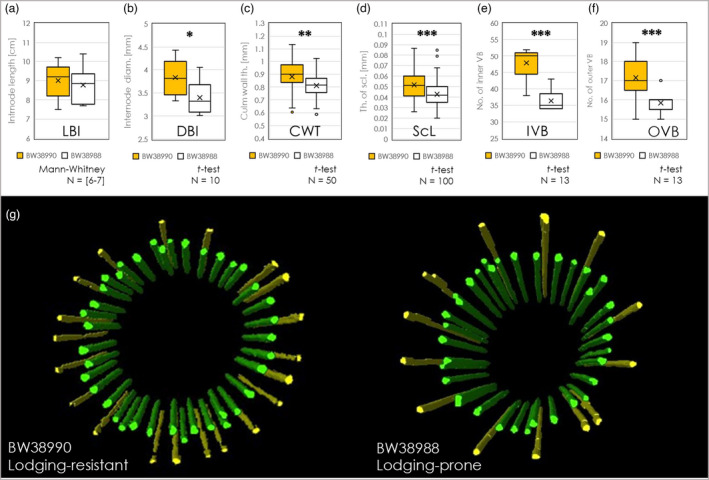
Comparative analysis of lodging‐resistant (BW38990—yellow bars) and lodging‐prone (BW38988—white bars) wheat lines. Lodging‐resistant (BW38990) and lodging‐prone (BW38988) wheat lines are characterized by similar length of the second basal internode (LBI, a). Nevertheless lodging‐resistant line’s internodes are significantly thicker than lodging‐prone internodes (DBI, b). Moreover, they are fortified with significantly thicker culm wall (CWT, c) and sclerenchymal layer (ScL, d), as well a higher number of inner (IVB, e) and outer vascular bundles (OVB, f), as it was observed in the ‘Stabilstroh’ rye line. The model of individual vascular bundles in inner (green) and outer vascular ring (yellow) in BW38990 and BW38988 based on 3D MRI data sets (g). Mann‐Whitney or *t*‐test; P* < 0.05, *P* < 0.01, P* < 0.001.
